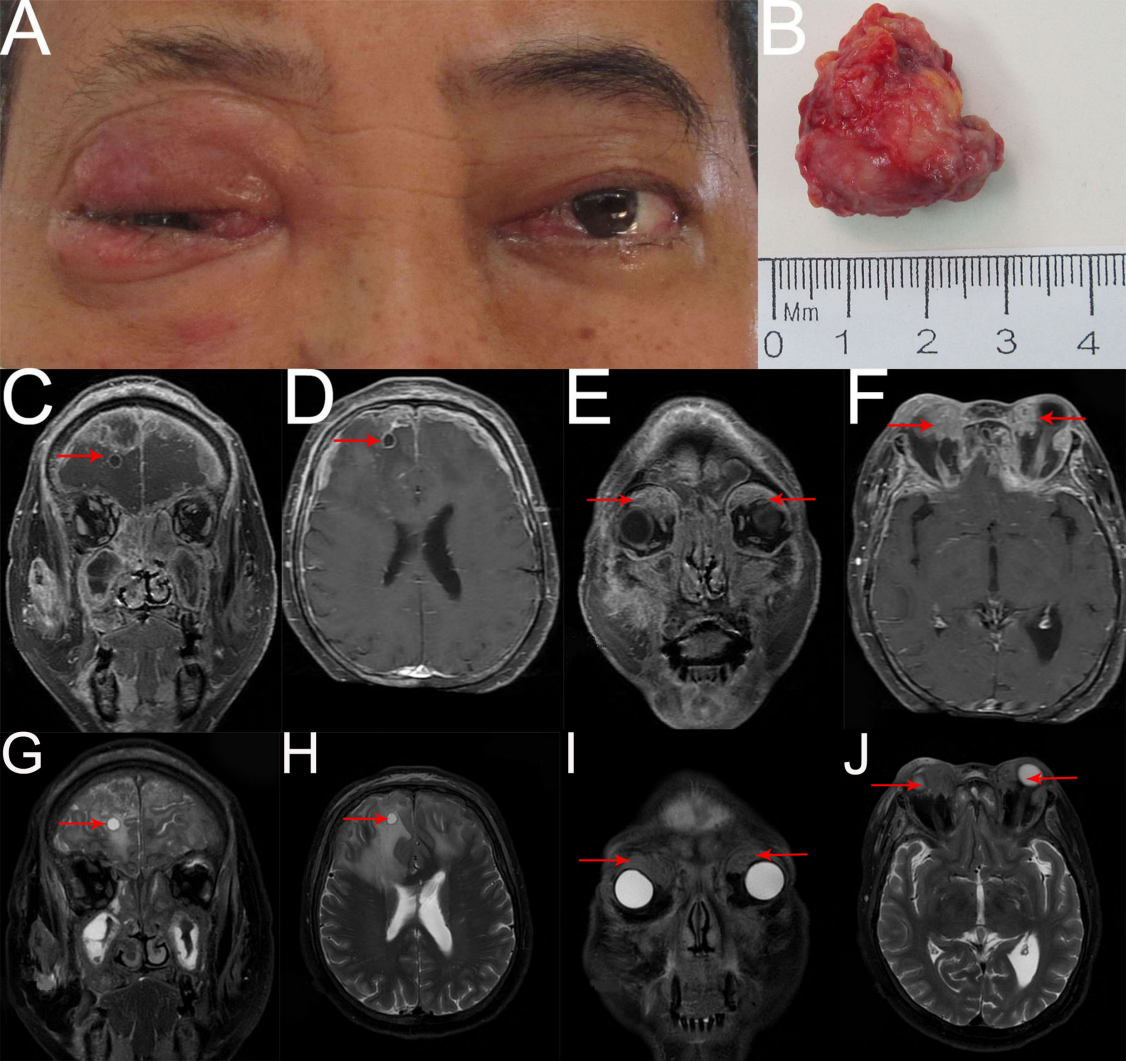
(A) External image of the second recurrence. (B) The mass was dissected under the table and was approximately 2.8cmx2.5cmx1.3cm in size. (C–J) Contract-enhanced MRI with STIR sequence of the orbit showed two irregular mixed-signal shadows on the right superomedial orbit and the left superior orbit with mild enhancement (E, F, I, J red arrow). Bilateral frontal meninges were thickened and enhanced obviously, and part of them had liquefaction necrosis. An analogous round mass with peripheral rim enhancement was seen in the right frontal lobe, and significant edema with unclear boundaries was observed in bilateral frontal lobes and corpus callosum (C, D, G, H red arrow). Additionally, numerous irregular abnormal signals with heterogeneous enhancement were observed in bilateral ethmoid sinuses, maxillary sinuses, sphenoid sinuses, frontal sinuses, right infratemporal fossa, face, zygomatic area, and parotid region, involving some bone.
